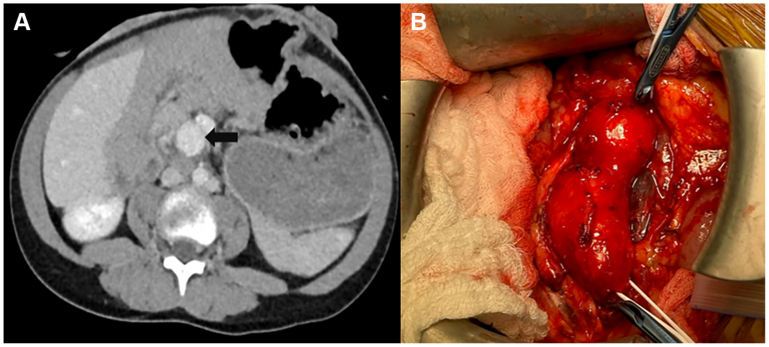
Shows a cross sectional computed tomography (CT) image, demonstrating a large superior mesenteric artery aneurysm (*black arrow*) (A) and intraoperative image of the aneurysm (B).
